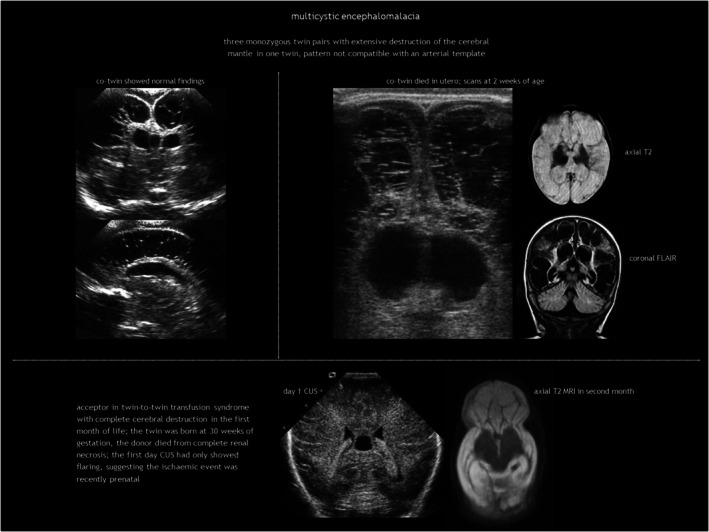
Multicystic encephalomalacia. Abbreviations: CUS, cranial ultrasonography; FLAIR, fluid‐attenuated inversion recovery; MRI magnetic resonance imaging.
